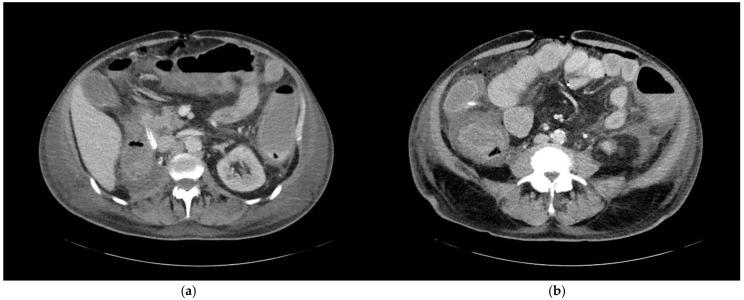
A 68-year-old patient with an unknown focus of origin. CT morphologic signs of colitis include (a) circular colonic wall thickening and pericolic fat stranding, and (b) decreased mural contrast enhancement indicating intestinal wall edema and adjacent ascites.
